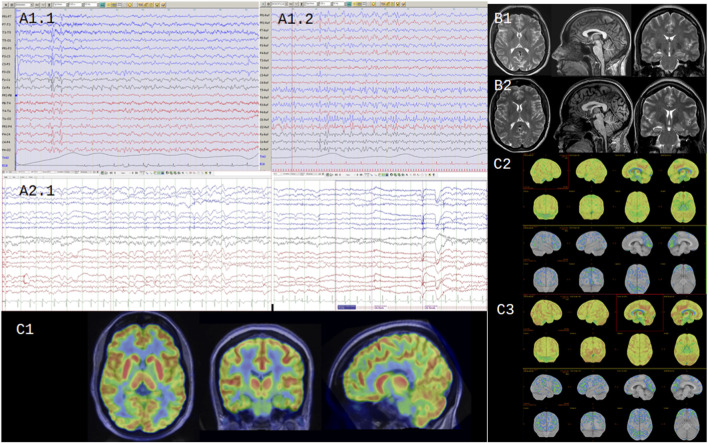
Neuroimaging and electrophysiological findings over time. (A1.1) EEG (2012, bipolar montage): generalized interictal epileptiform discharges with maximum electronegativity in the occipital regions. (A1.2) EEG (2012): intermittent photic stimulation elicits occipital driving response. (A2.1) EEG (2025, bipolar montage): no interictal epileptiform discharges observed throughout the recording; intermittent photic stimulation produces no changes. (B1) Brain MRI (2012 and 2016): no significant changes observed between the two studies. (C1) FDG-PET/MRI (2023): performed 30 min after intravenous injection of 4 mCi of [18F]-FDG using a Signa GE PET/MRI scanner; moderate hypermetabolism observed in the basal ganglia and thalami in axial (A), coronal (B), and sagittal (C) views. (C2) Quantitative PET analysis (2023): automatic 3D-SSP analysis with normalization to pontine activity identifies regions of statistically significant hypometabolism (compared to age-matched controls), shown in yellow and red on three-dimensional reconstructions. (C3) Quantitative PET analysis after 1 year of metformin treatment (2025): expanded areas of hypometabolism, predominantly in the frontal lobes.
